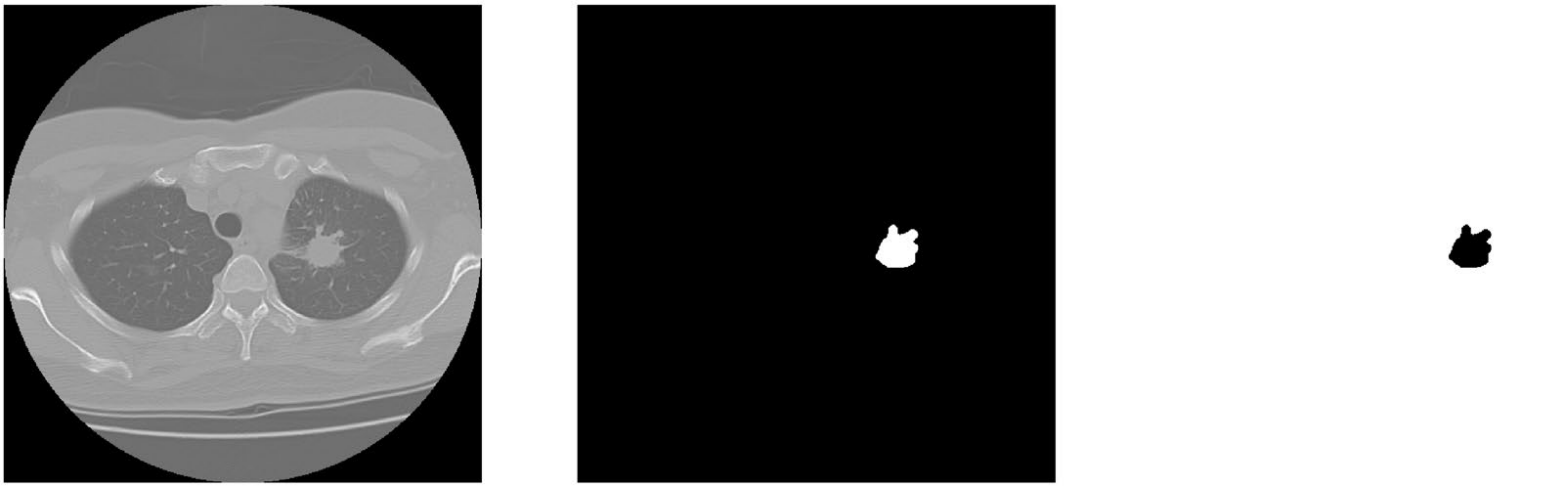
Inverting the pulmonary label. From left to right, CT image, original label, complementary label.
